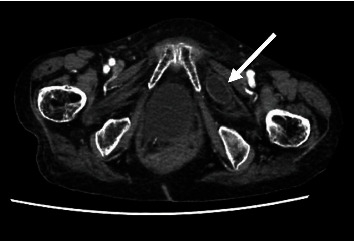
Axial contrast-enhanced computed tomography: representative image demonstrating a segment of small bowel protruding through the obturator canal (white arrow).
